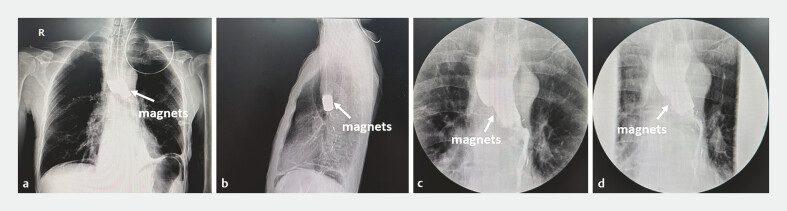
Postoperative X-ray monitoring by esophagography:
